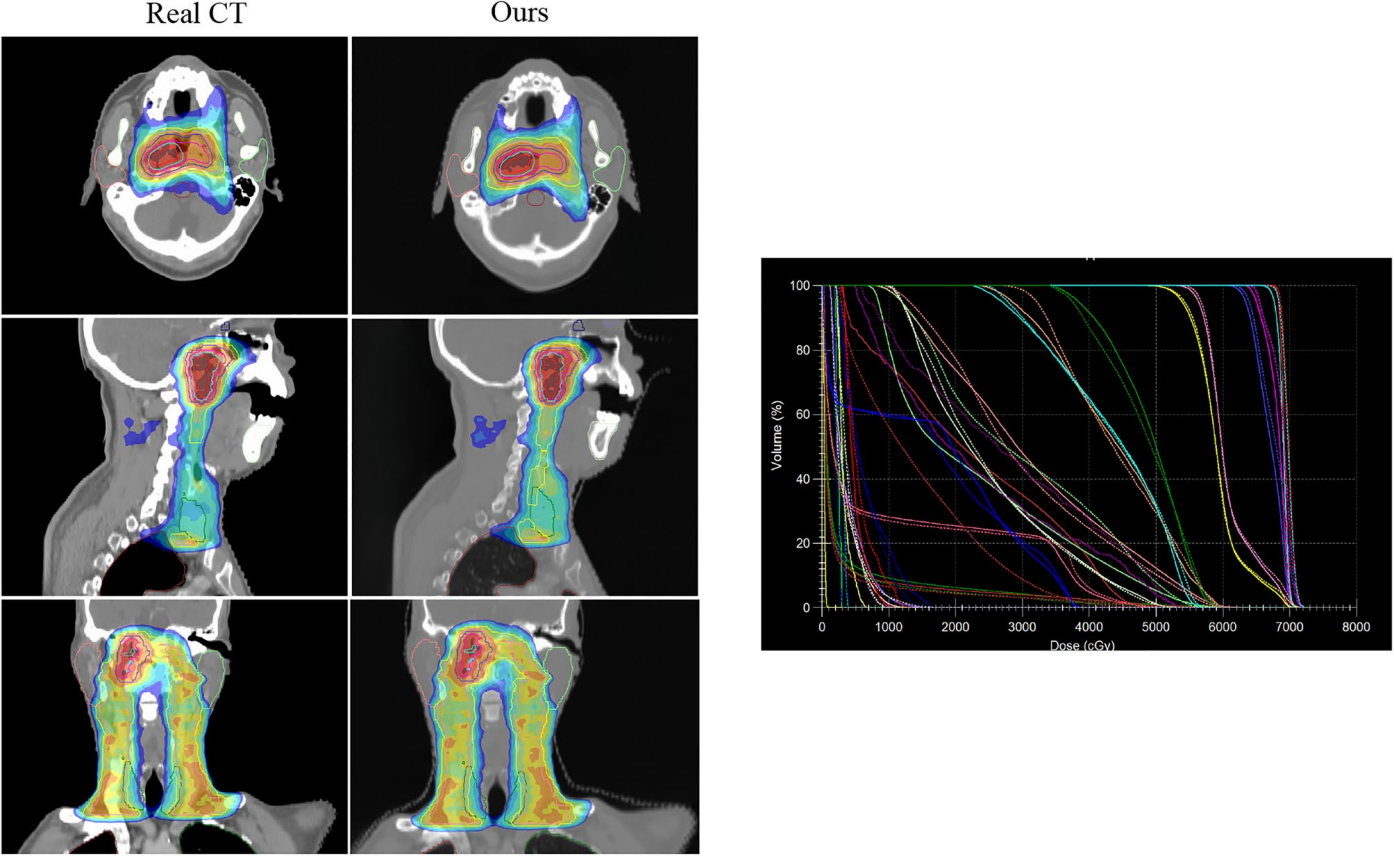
3D reconstruction visualization of the H &N dataset. From top to bottom, representing axial, sagittal and coronal planes(left). Patient DVH differences, where the solid line is Real CT and the dashed line is sCT (right).
